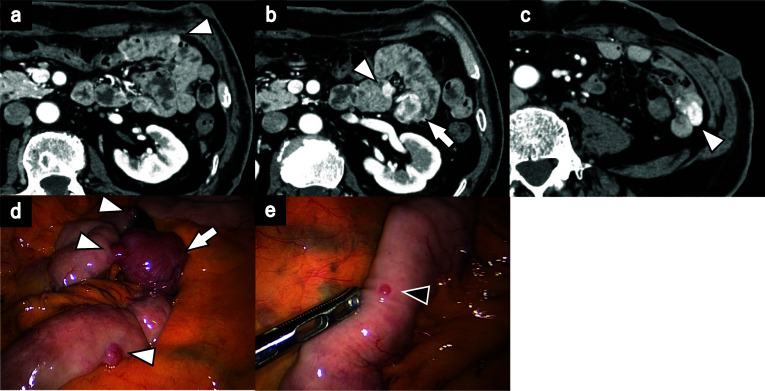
a–c Contrast-enhanced computed tomography findings. Axial image. Multiple contrast-enhancing masses were seen in the small intestine (white arrowhead). The largest mass was approximately 20 mm in diameter (white arrow). d, e Intraoperative findings. Multiple masses were seen in the small intestine (white arrowhead), with the largest mass located in the upper jejunum (white arrow). Multiple small lesions less than 5 mm in size were also seen (black arrowhead)
